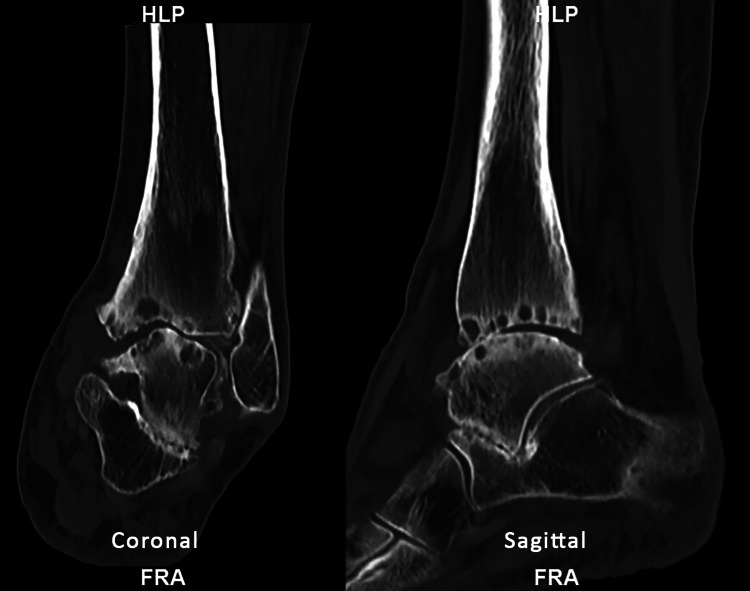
Coronal and sagittal CT scans of the left ankle Coronal and sagittal CT scans of the left ankle demonstrate significant osteoarthritic changes with subchondral cysts noted on tibia, talus, and calcaneus with sclerosing of the tibiotalar and subtalar joint surfaces. There is varus hindfoot and talar tilting noted.
